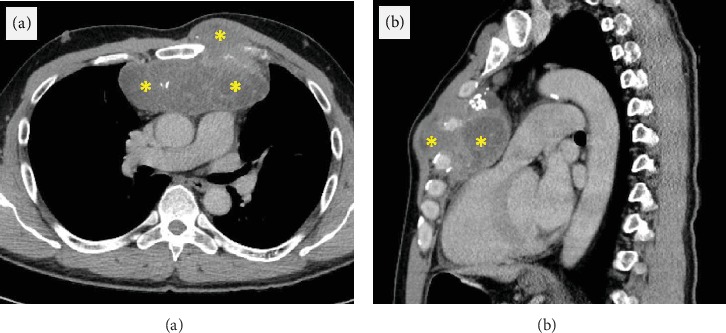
Chest wall chondrosarcoma on prior CT scan. CT scan of chest wall chondrosarcoma in (a) transverse and (b) sagittal planes marked by asterisks.
